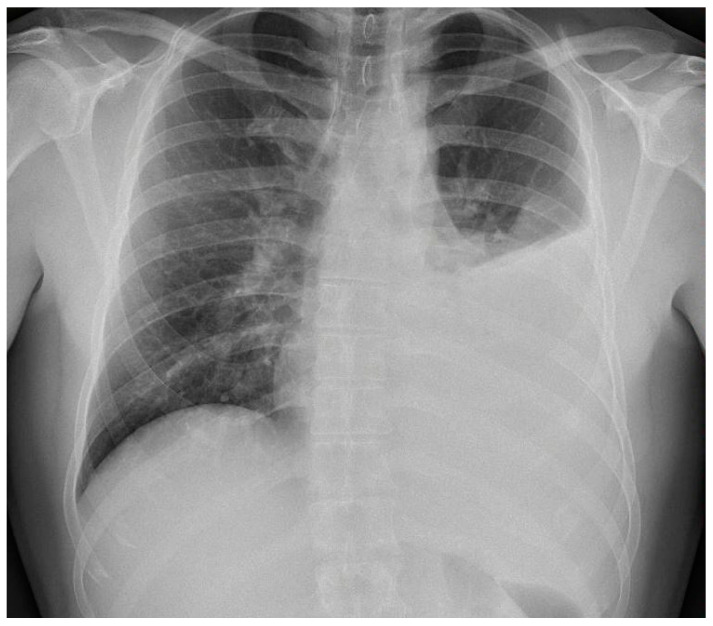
Chest X-ray during second admission to the ward revealed fluid in the left pleural cavity reaching the third rib.
